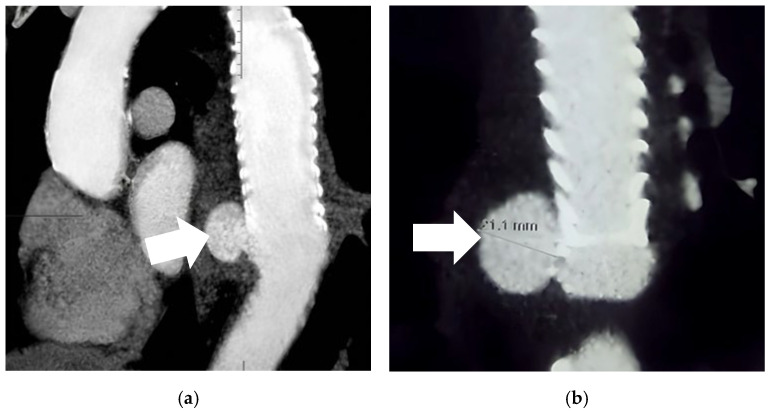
CTA demonstrating a 21 mm aneurysmal lesion of the descending thoracic aorta without contrast extravasation or endoleak (arrow) (a) with ×2 magnification and measurement indicated (b).
